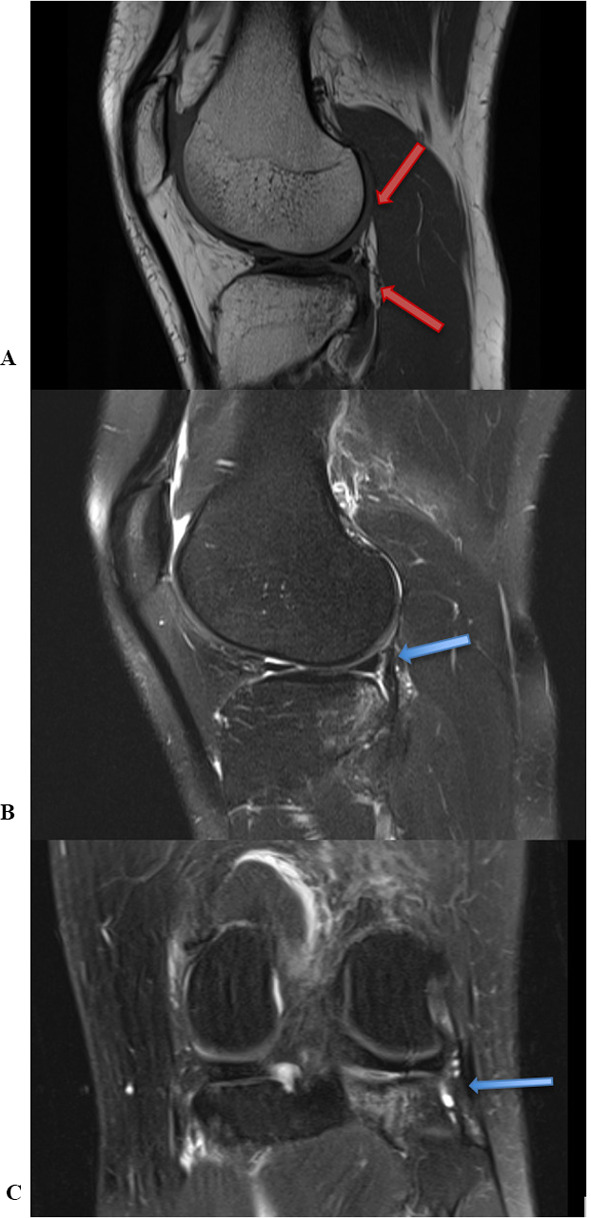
A, B and C Pre-operative MRI. Sagittal (Fig. 2A, B) and coronal (Fig. 2C) views of popliteomeniscal fascicle tears. The red arrows indicate the tear of antero-inferior and postero-superior bundles of PMFs on the sagittal view in T2-weighted sequences. The blue arrow demonstrates the PMF tear in T1 sequences on the sagittal view
